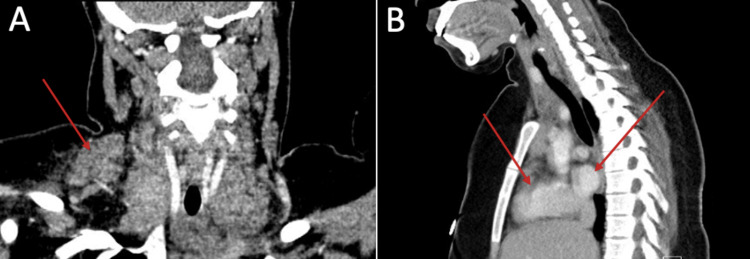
CT scan of an 18-year-old female showing multiple enlarged lymph nodes. A: Coronal cut of the CT scan showing enlarged right supraclavicular lymph node. B: Sagittal cut of the CT scan showing multiple enlarged lymph nodes in the aortopulmonary window and paratracheal region.
